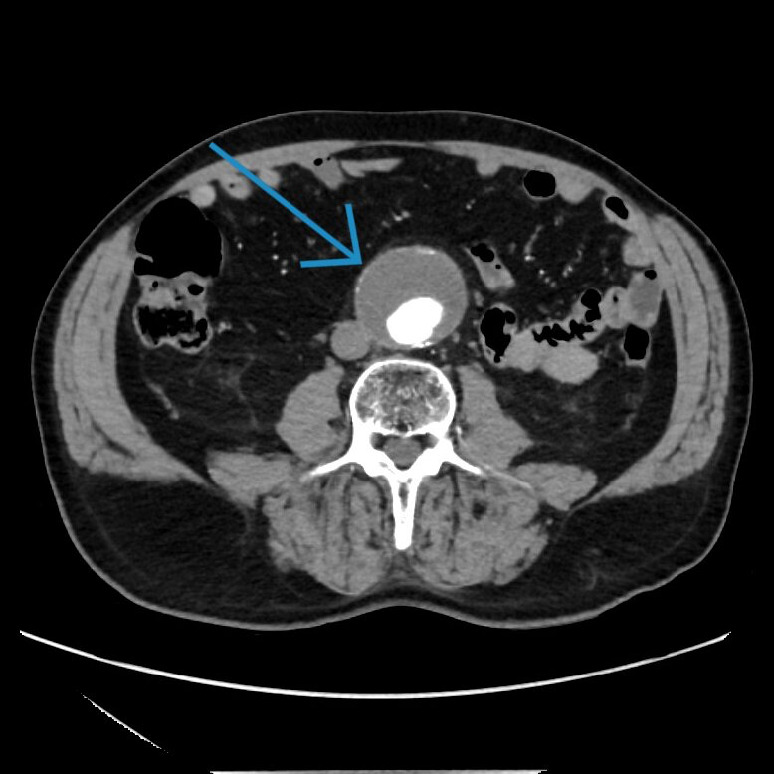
Computed tomography (CT) angiogram of the chest, abdomen, and pelvis demonstrating a 5 x 5.3 cm infrarenal aortic aneurysm extending to the aortic bifurcation (8.9 cm long) and a 1.3 cm calculus at the left ureteropelvic junction, resulting in moderate hydronephrosis.
